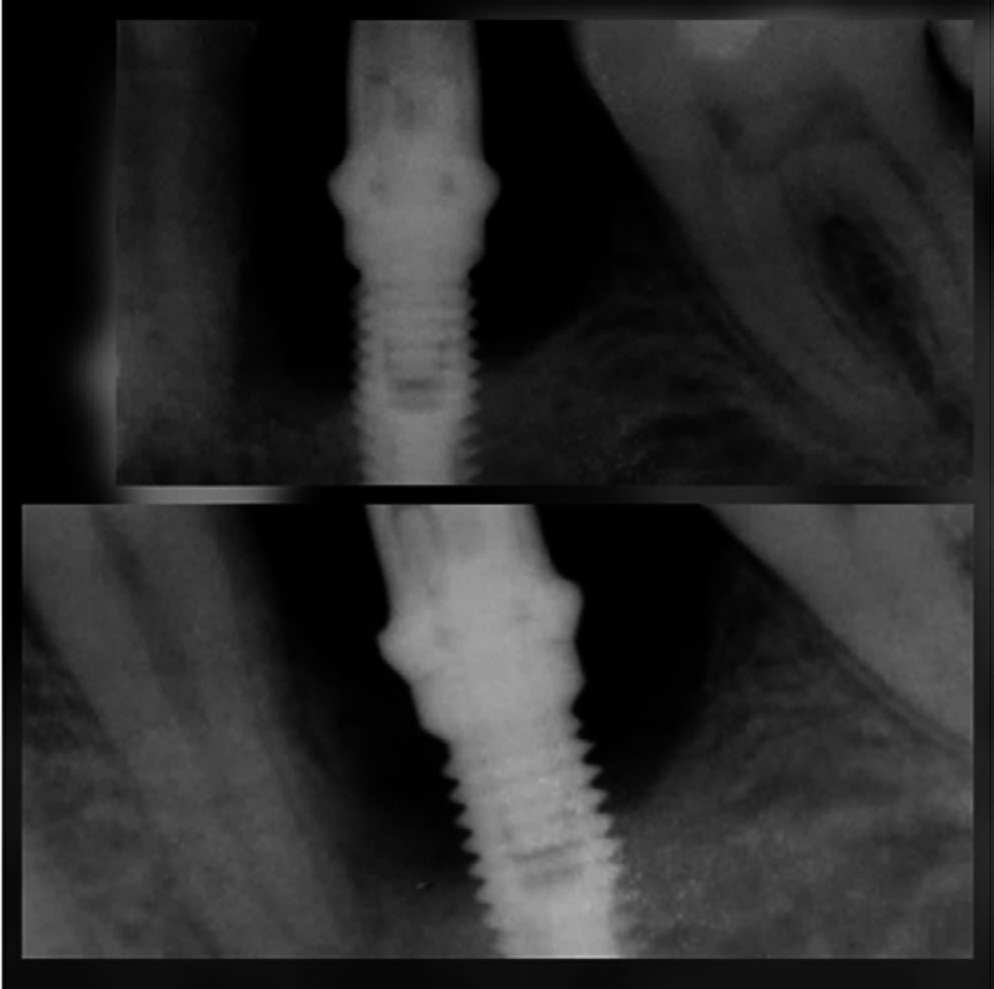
Intraoral periapical radiograph of implant site 2 mo after treatment
